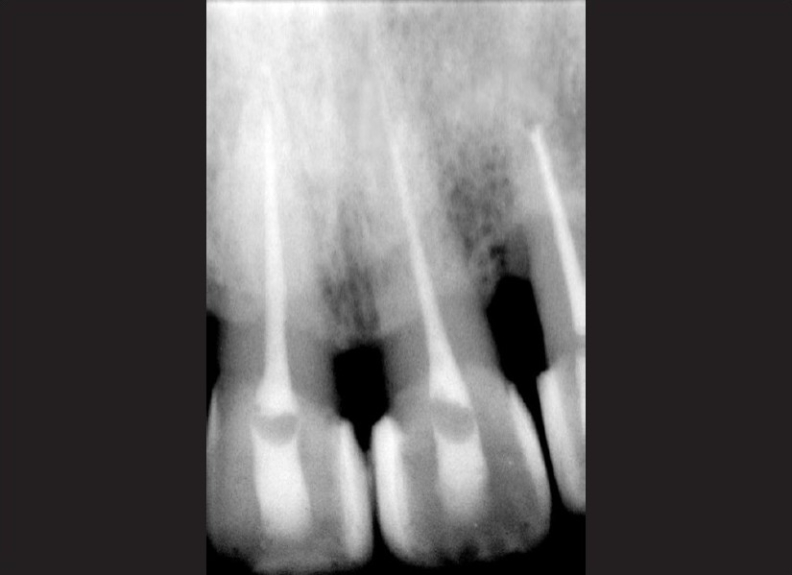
IOPA radiograph at 2-year follow up
